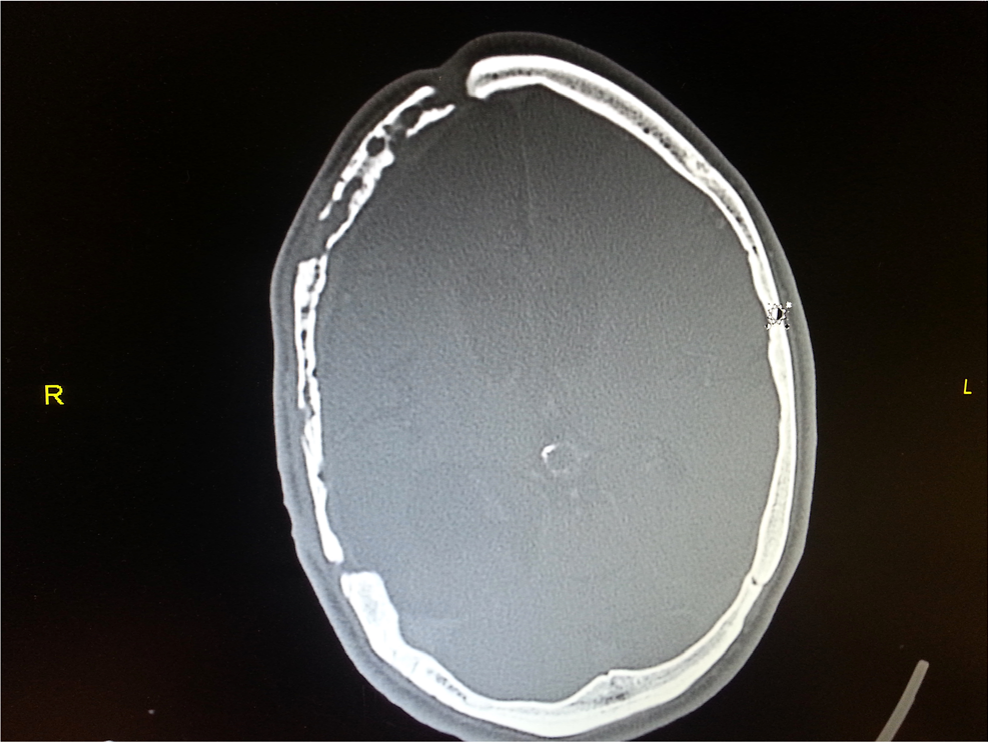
Bone flap resorption on CT scan
